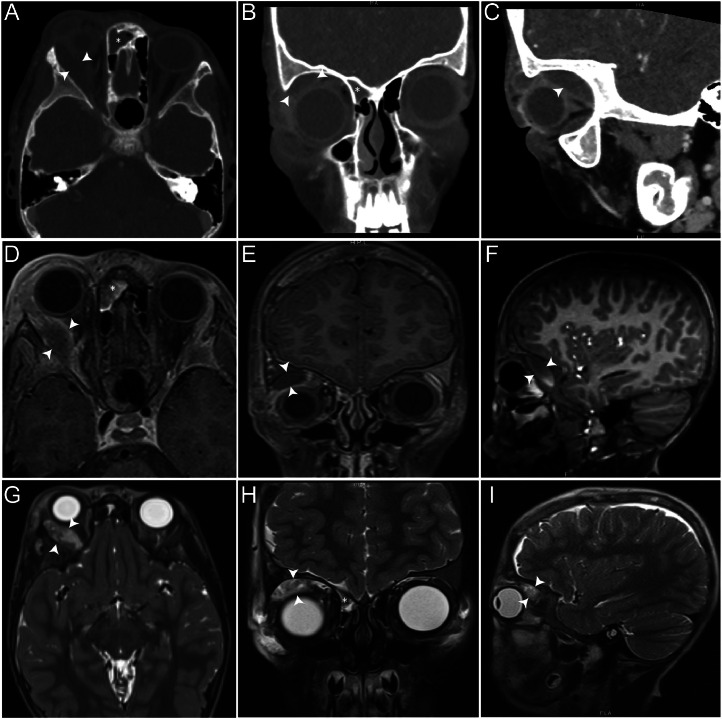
Contrast-enhanced CT and MRI imaging of a child with sickle cell disease presenting with fever, unilateral proptosis, and periorbital swelling. Contrast-enhanced CT of the orbits and paranasal sinuses in axial (A), coronal (B), and sagittal (C) views: White arrowheads in each panel delineate the extent of a right-sided subperiosteal fluid collection measuring ∼1.2 cm in greatest dimension, along the superior and lateral orbital walls, resulting in proptosis and hypoglobus, evident in (B). This mass effect causes compression and medial displacement of the extraocular muscles, particularly the superior rectus and lateral rectus muscles. The black asterisk in (B) shows near-complete opacification of the right ethmoid sinus with a corresponding white asterisk indicating the adjacent medial orbital wall and preseptal swelling. The globe is anteriorly displaced and the retrobulbar space is narrow secondary to the posterior extension of the subperiosteal collection. T1-weighted MRI in axial (D), coronal (E), and sagittal (F) views, and T2-weighted counterparts in (G-I). White arrowheads point to the subperiosteal fluid collection, which appears hypo- to isointense on T1 and hyperintense on T2 sequences. The asterisk in (H) indicates the opacified contiguous right ethmoid sinus with air-fluid levels. These T2-weighted images also clearly demonstrate the multiloculated nature of the subperiosteal fluid collection, with internal septations visible in the coronal view (H, white arrowheads). The loculation, while concerning for abscess formation, can also be seen in organizing hematomas as fibrovascular tissue develops within the cavity. The lack of significant soft tissue edema or infiltration further supported hematoma over infectious etiology, though this distinction remained challenging given the clinical context of fever and elevated inflammatory markers.
